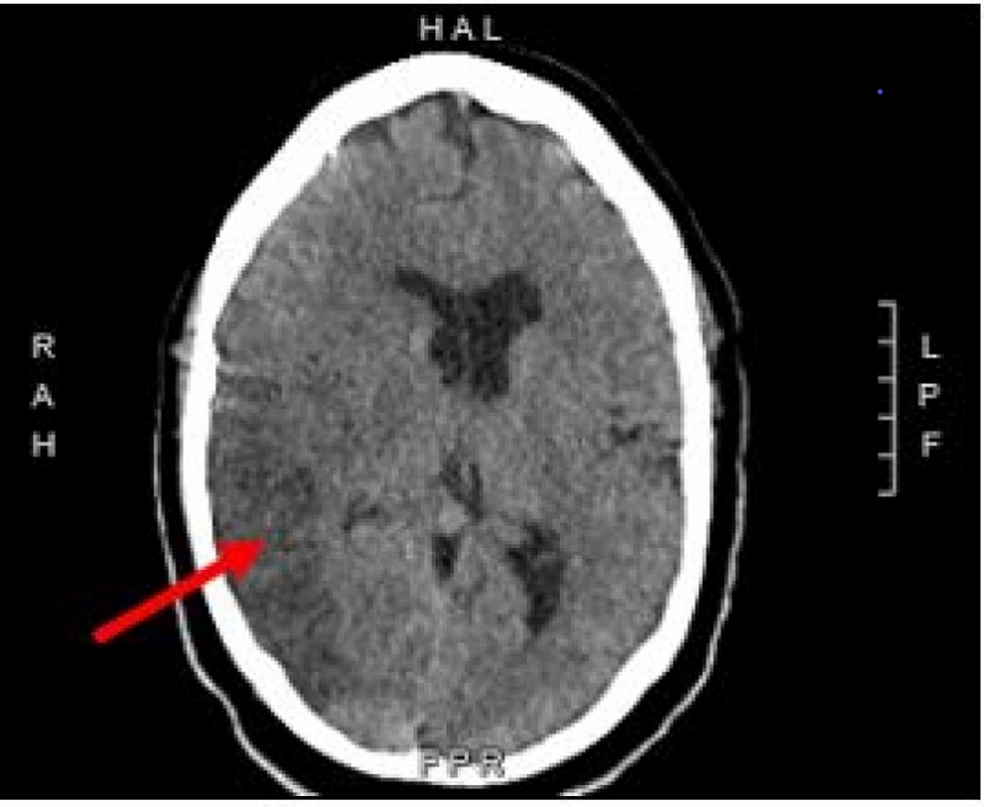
Noncontrast head CT demonstrating a large infarct involving the right MCA vascular distribution with mass effect. MCA, middle cerebral artery
